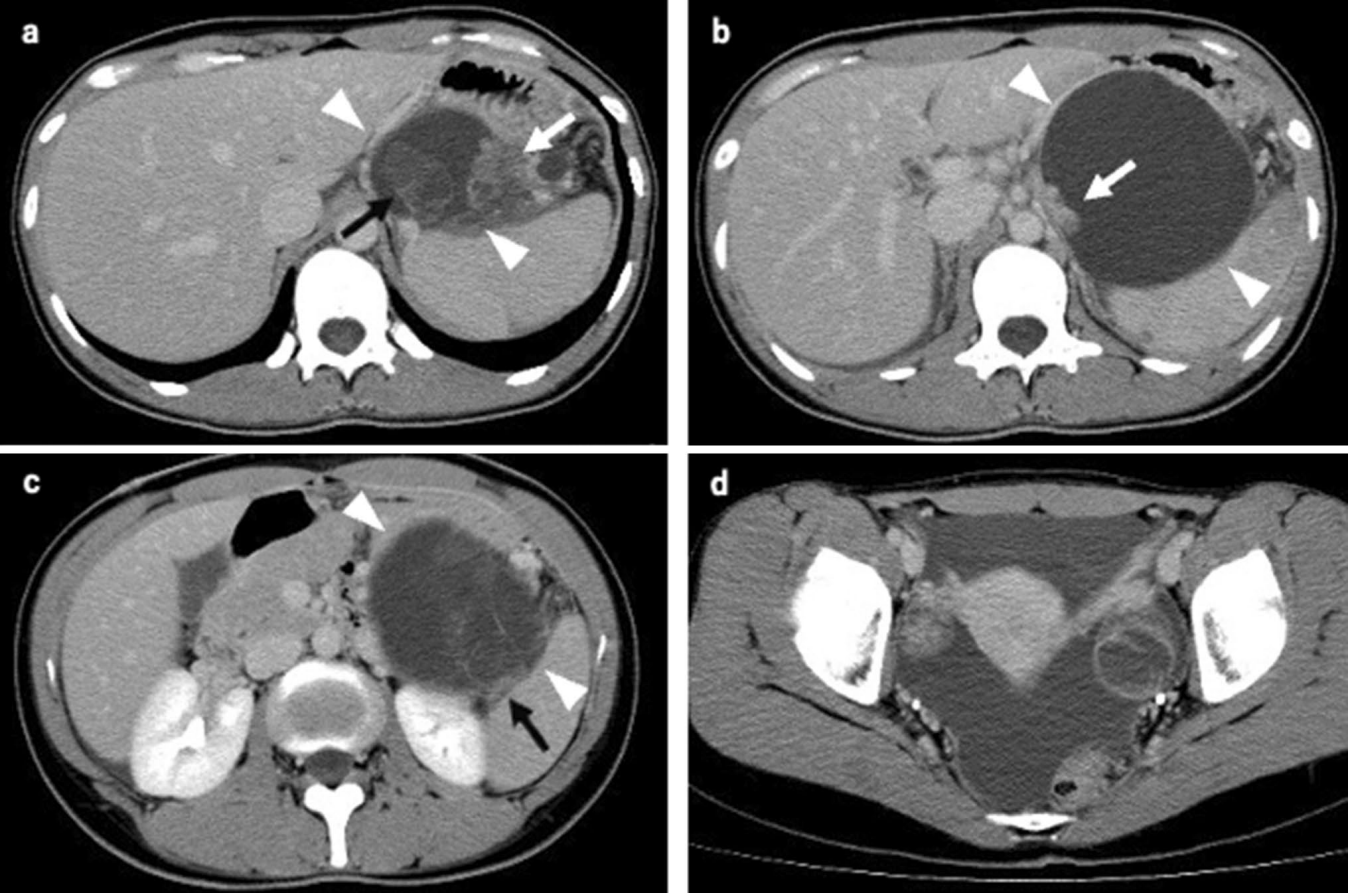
A 28-year-old woman with a mucinous cystic neoplasm of the pancreas. Contrast-enhanced computed tomography shows a well-defined 9.5 × 6.4 cm multilocular cystic lesion (white arrowhead) in the tail of the pancreas and ascites in pelvis. The cystic lesion had a cystic component (black arrow) and a mural nodule (white arrow) along the cyst wall
